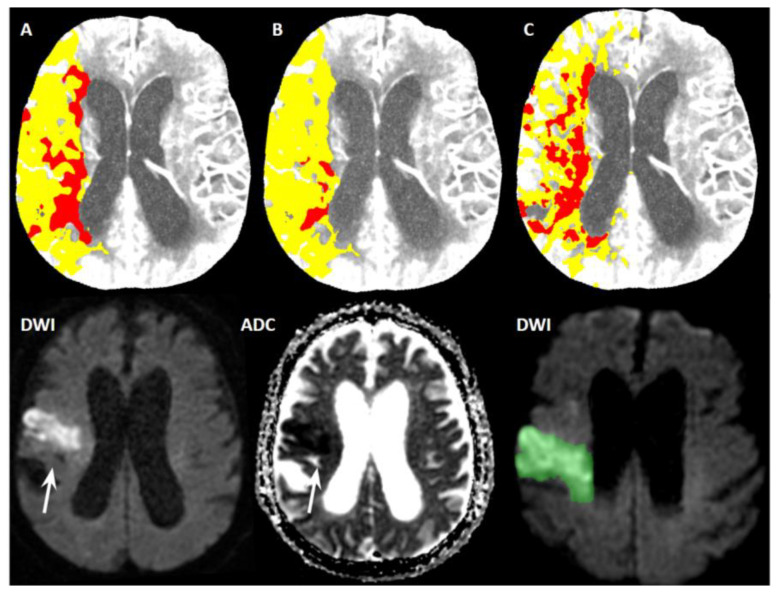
Comparison of three different syngo.via software settings with final infarct volume on magnetic resonance imaging follow-up in an exemplary patient. A 75-year-old man with acute ischemic stroke due to right middle cerebral artery occlusion confirmed on CT angiography. CT perfusion maps (upper row) obtained using different syngo.via software settings depicting the different extent of ischemic core (red area) and penumbra (yellow area). Using Setting A, the ischemic core was 35 mL (A), for Setting B, the ischemic core was 6 mL (B) and for Setting C, the ischemic core was 54 mL (C). Magnetic resonance imaging follow-up (lower row) 24 h after endovascular treatment depicted the final infarct as a hyperintense area on diffusion-weighted images (DWI) with a hypointense area on an apparent diffusion coefficient (ADC) map (arrow). Final infarct volume (green area) measured on magnetic resonance imaging was 29 mL.
